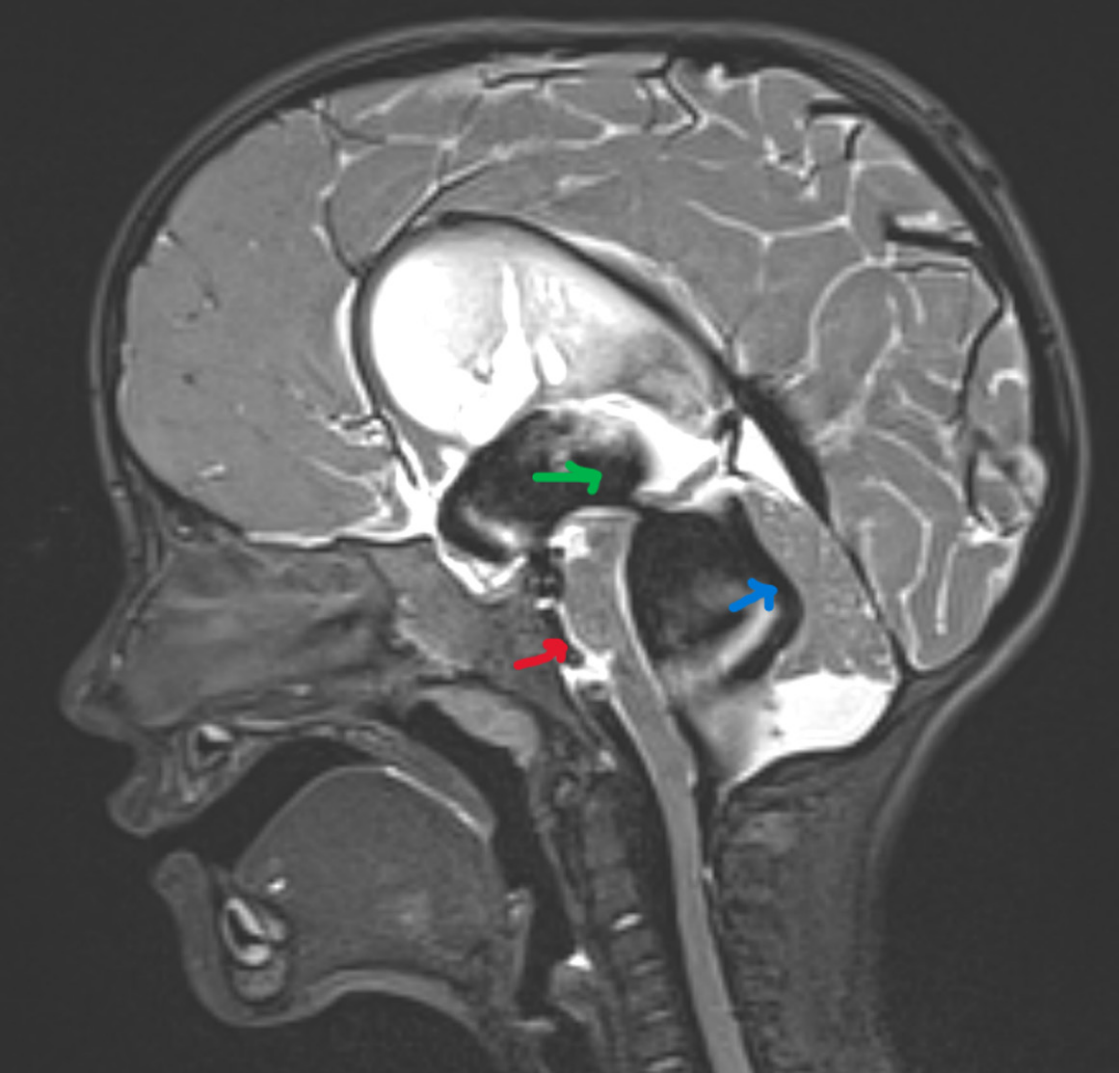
T2 sagittal MRI imaging of Case 1 shows a normal position of the brainstem (red arrow) and regression of the compression of the cerebellum (blue arrow) 1 week after ETV with flow void through the ventriculostomy and the aqueduct (green arrow).
